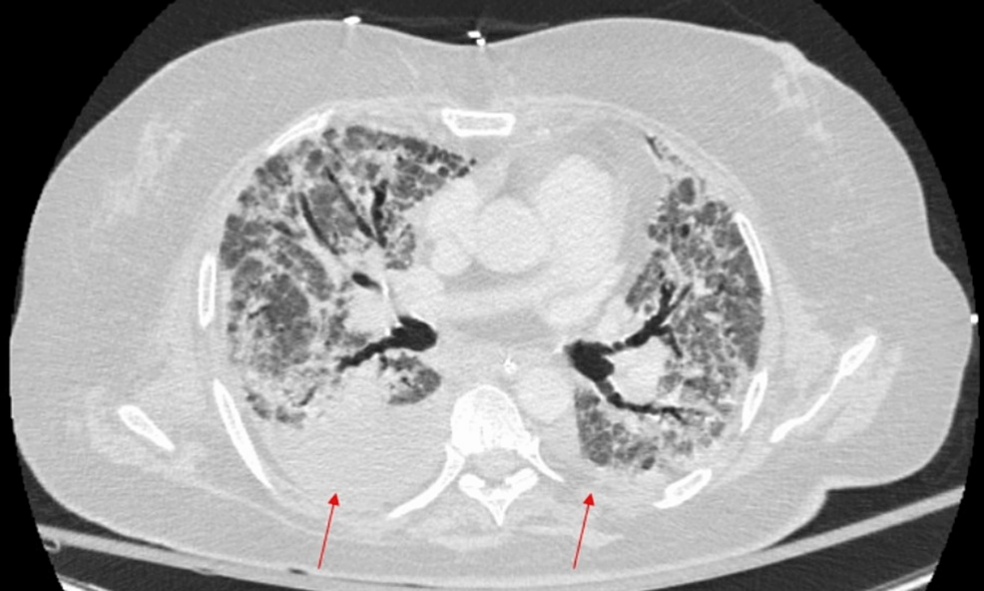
CT thorax with contrast showing lung fibrosis and moderate right and small left pleural effusion (red arrows).
